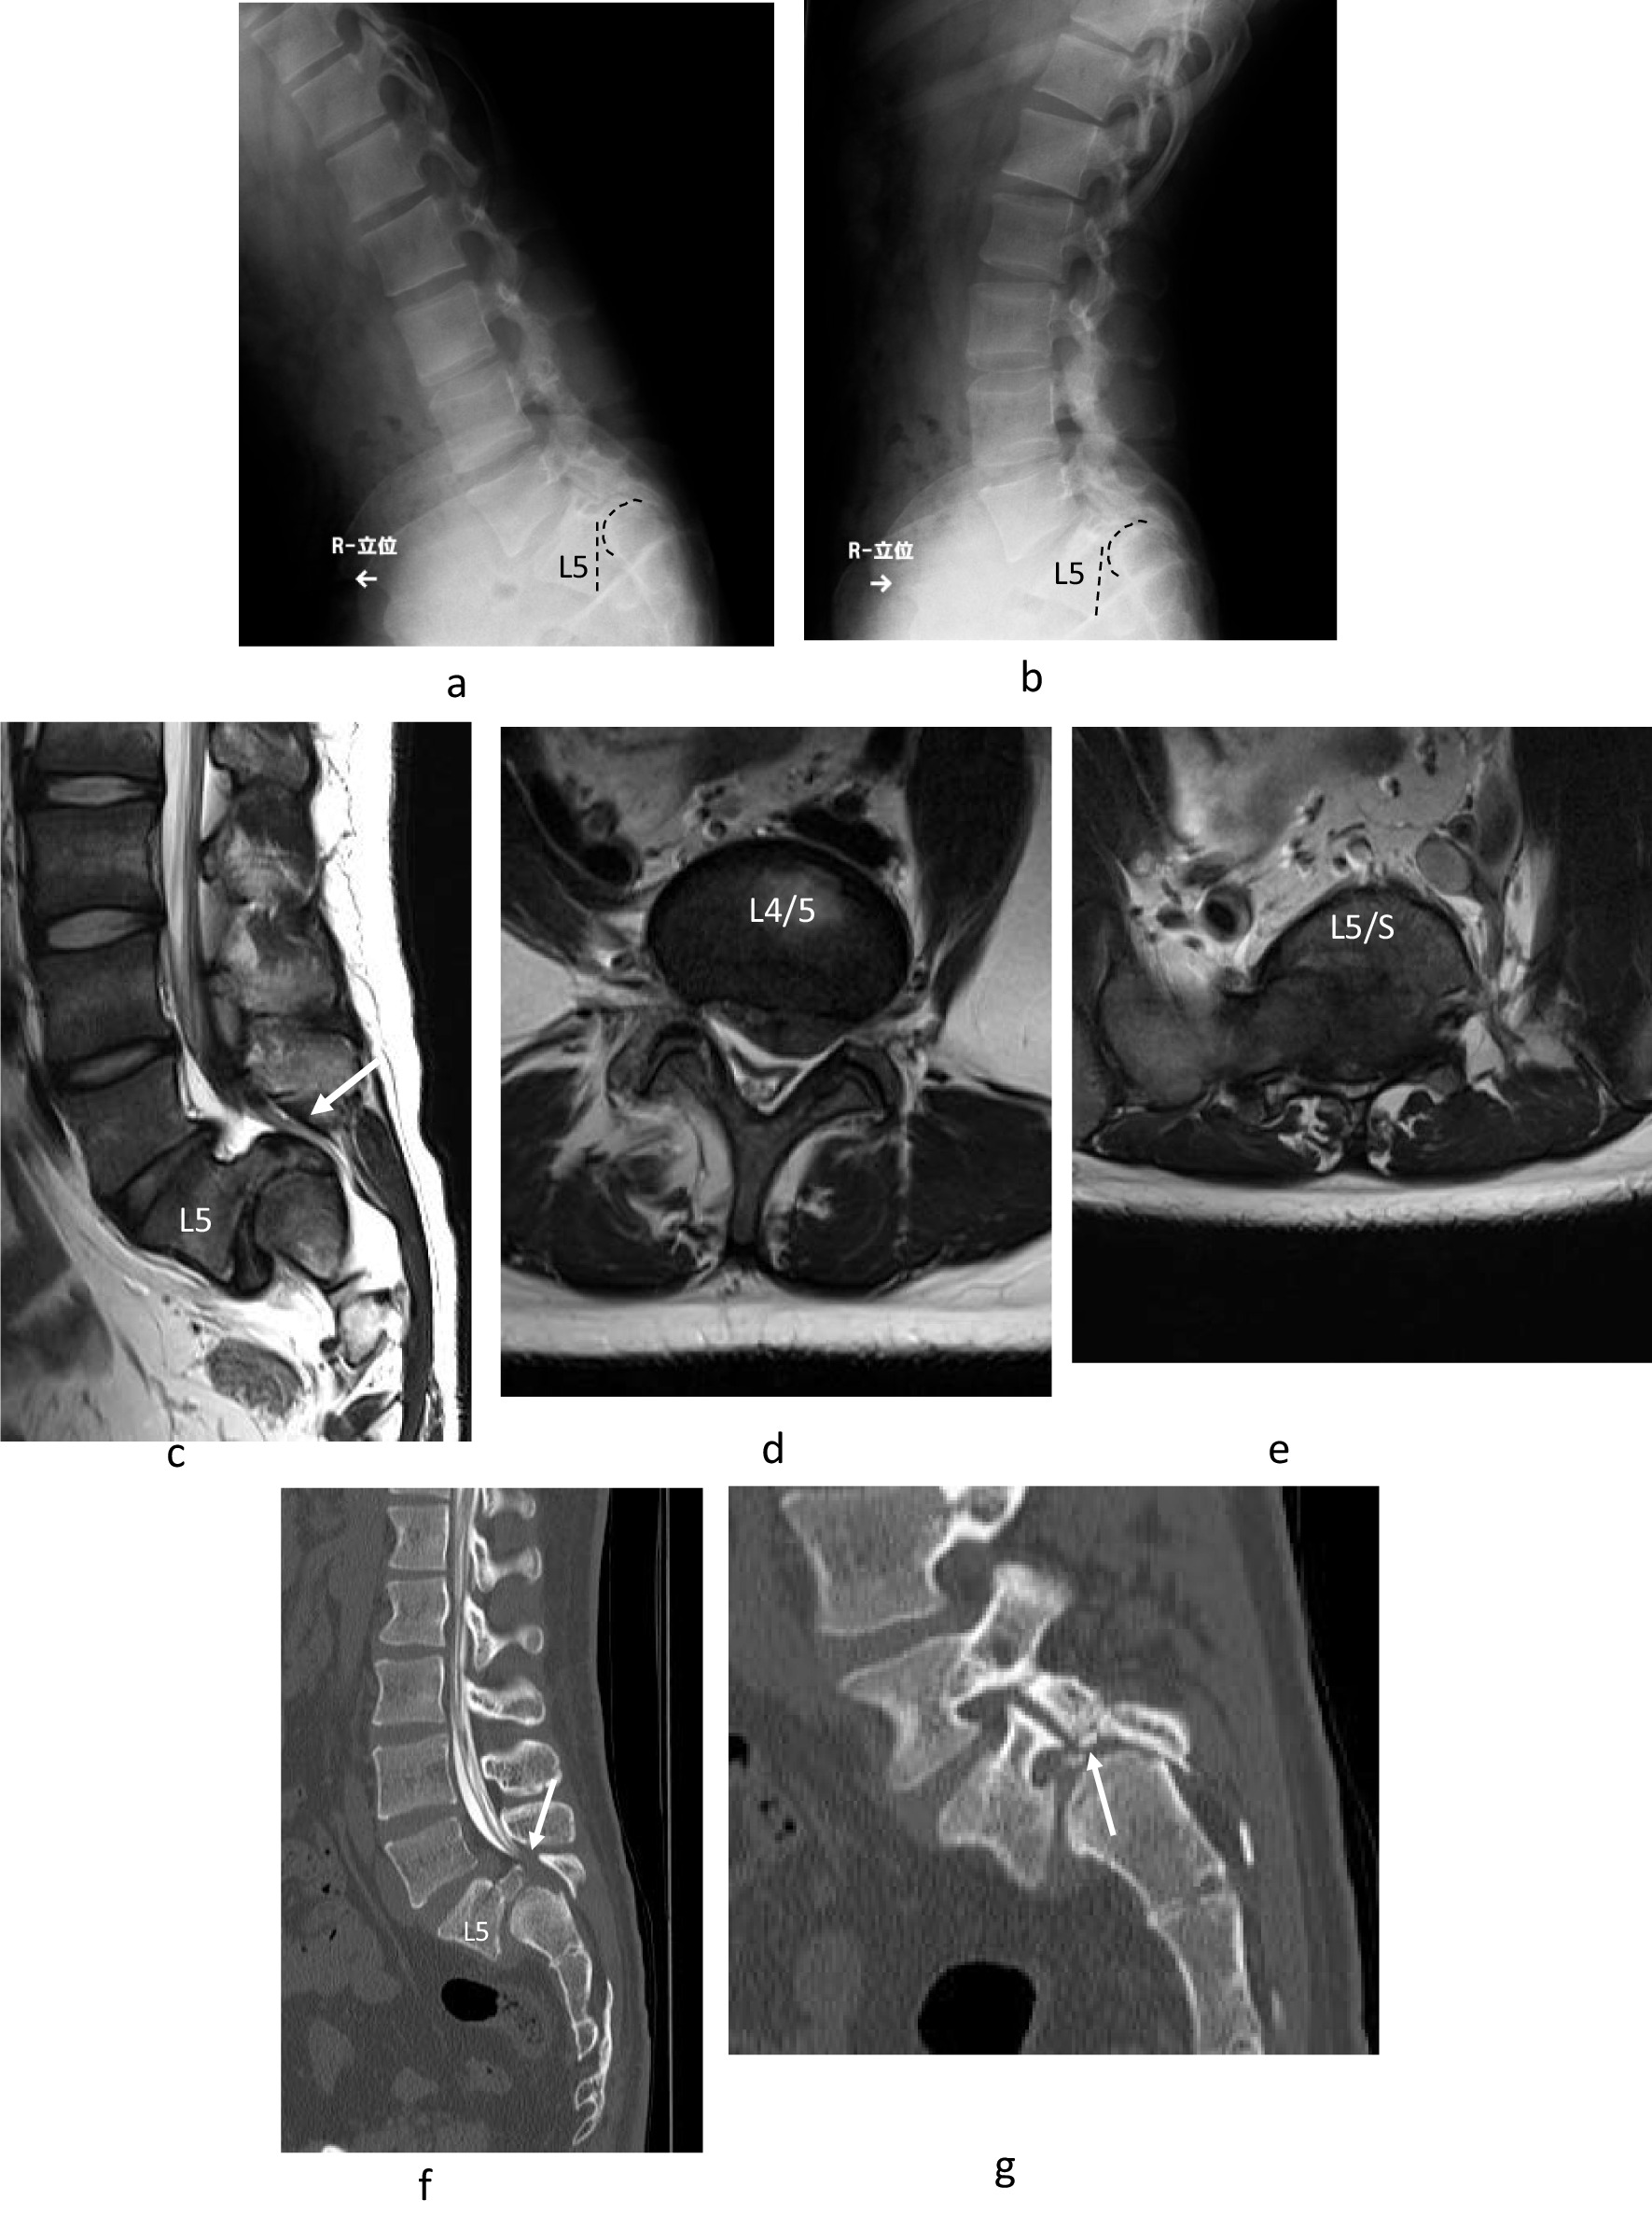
Lateral-view functional plain X-ray in the younger brother showing slight instability between L5 /S1 flexion and extension (a, b). MRI revealed that the L5 vertebra had slipped anteriorly on S1 and canal stenosis was present at L4/5 and L5/S1 with disc bulging (c–e). Computed tomography with myelogram showed a vertically displaced anterior slip to S1, a round-shaped S1 cranial endplate and spinal canal stenosis (arrow) with dysplastic bilateral facets at L5/S1 and unilateral pars defect of the right inferior articular process of L5 (arrows) (f, g)
